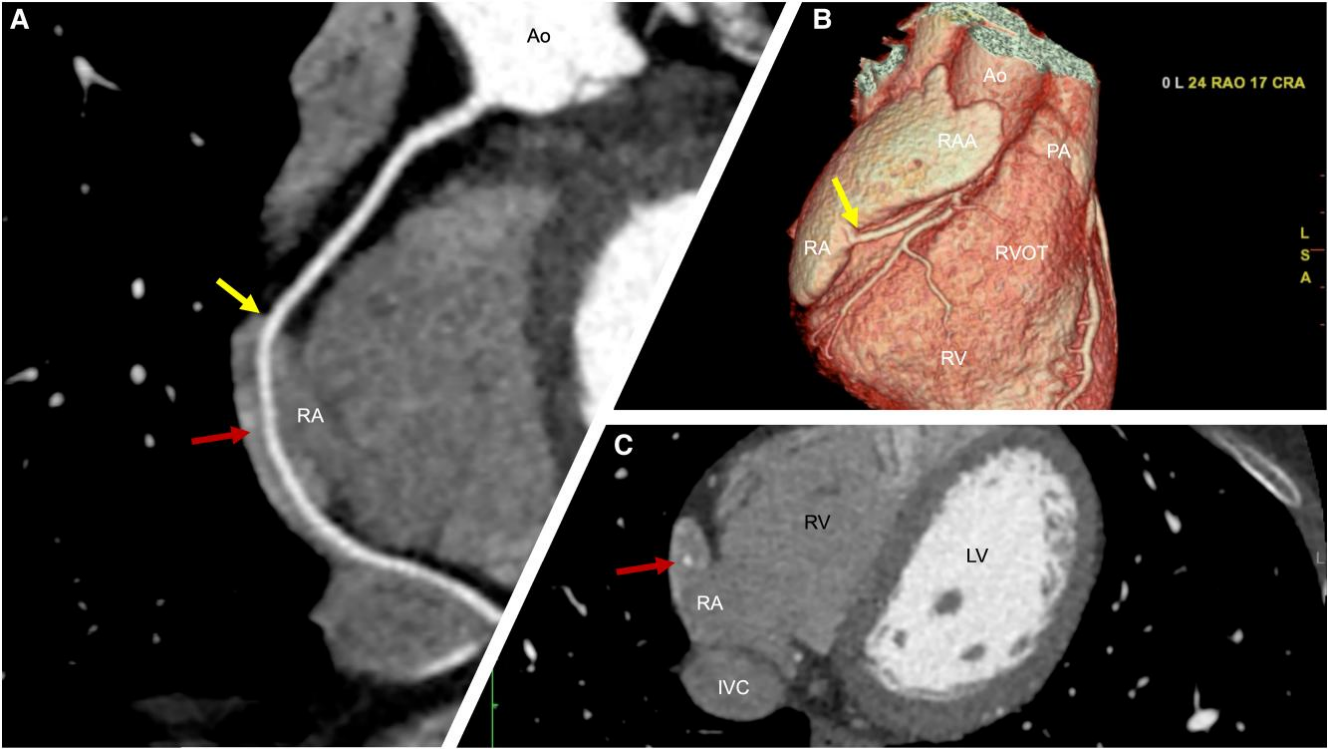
A 48-year-old woman with no relevant past medical history was referred for coronary computed tomography angiography (CCTA) due to complaints of atypical angina lasting for 2 months.
